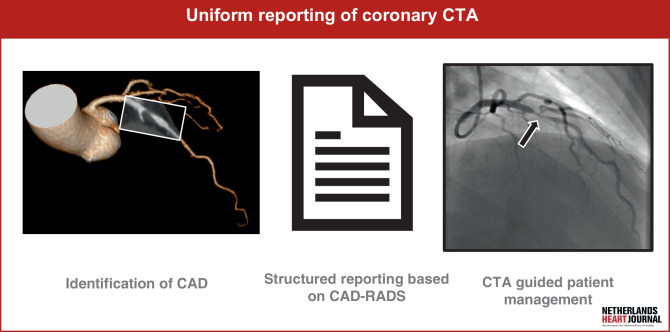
Infographic: Uniform reporting of coronary CT
